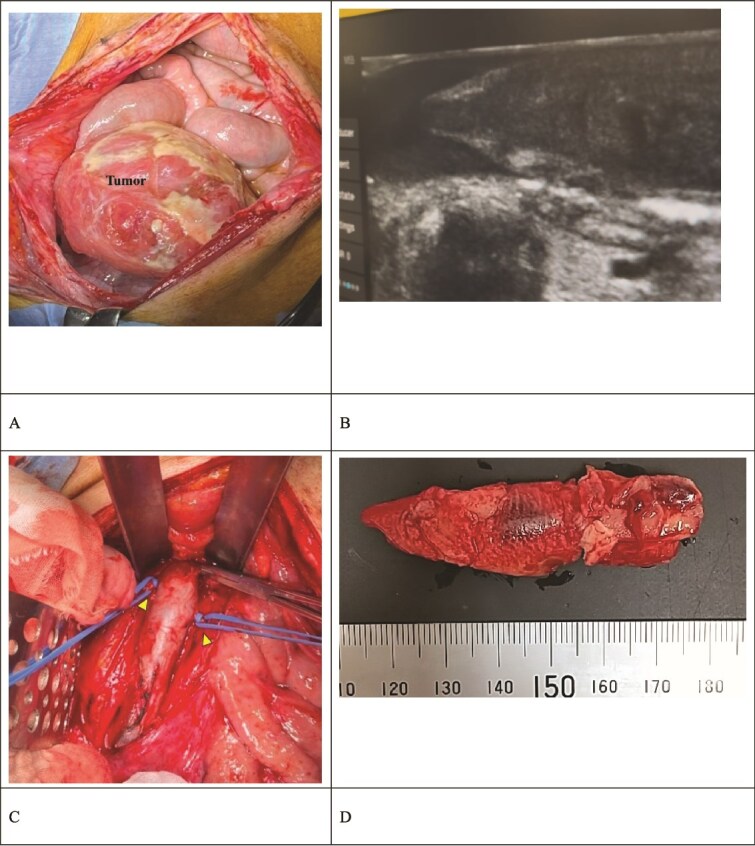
Intraoperative findings. (A) The ovarian tumor did not adhere to the peritoneal cavity. (B) Intraoperative ultrasound revealed a thrombus extending to the IVC below the renal vein. The thrombus had high echogenicity at the periphery and low echogenicity internally. (C) The IVC was clamped below the renal vein. The bilateral ovarian veins were ligated with vessel loops (arrow). (D) The extracted thrombus measured 65 × 15 mm and was already organized.
